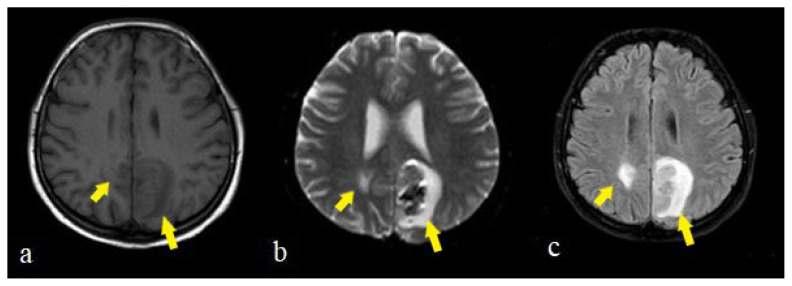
The brain MRI of a 28 years old female patient showed bilateral parieto occipital cortical hypointensity on T1W (a), hyperintensity on T2W (b), and FLAIR (c) images with hemorrhagic transfromation on the left (b) (arrows).
